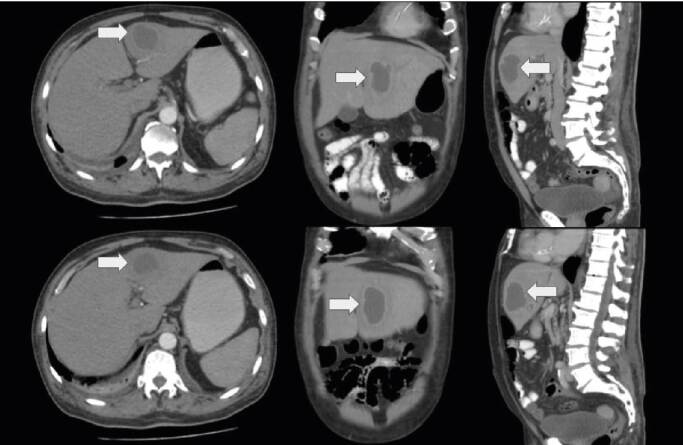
Computed tomography of abdomen and pelvis with intravenous contrast. The arrow shows a variable density internal pattern of a hypodense lesion in relation to the liver parenchyma, compatible with an abscess in the left hepatic lobe.
